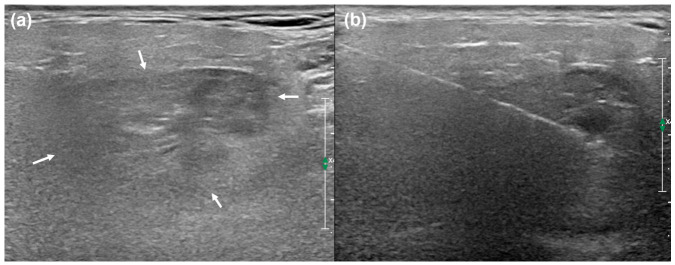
US findings. (a) Ultrasound shows a heterogeneously hypoechoic mass in the right parotid gland (arrows), with limited evaluation of the deep portion due to limited acoustic penetration. (b) An ultrasound-guided core-needle biopsy was performed targeting the fat component of the mass in the superficial lobe. The biopsy needle is visualized as a linear echogenic line in the image.
